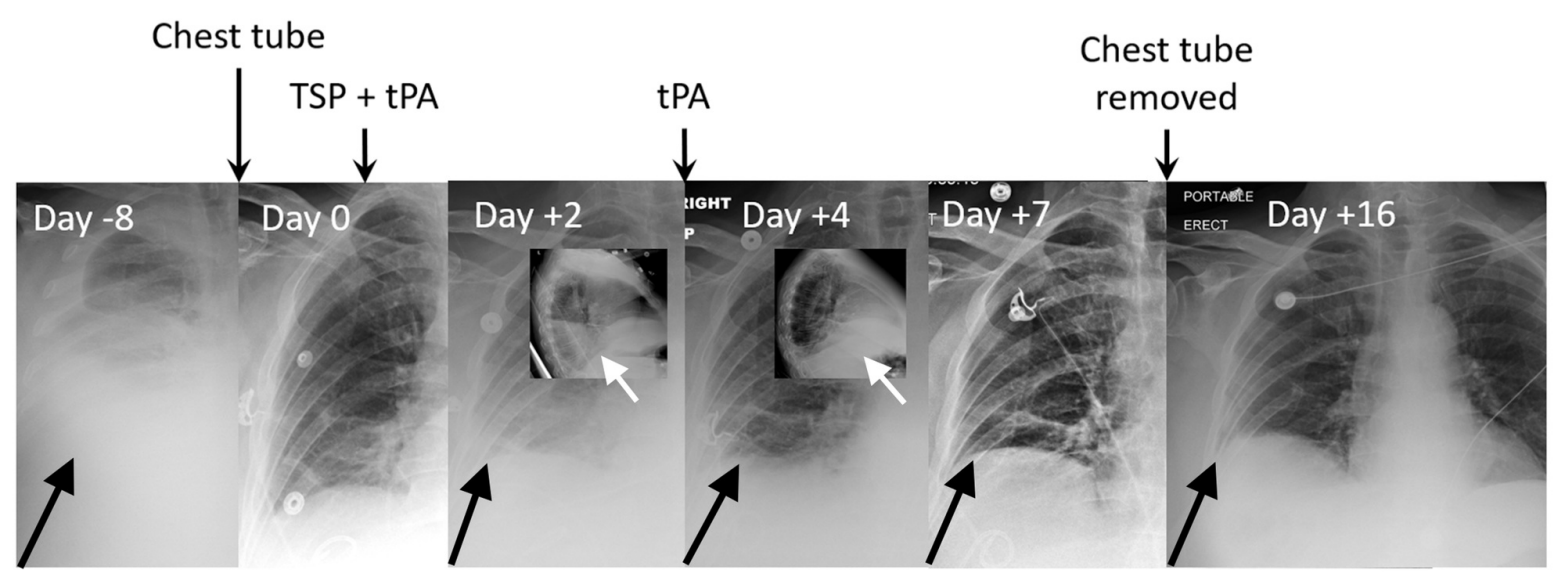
tPA after TSP for drainage of developing loculation. A 67-year-old man presented with hepatic hydrothorax (day -8). A pigtail chest tube was placed with output >2,000 cc/day. TSP with tPA, 6 mg, was given on day 0. Lateral chest x-ray on day +2 demonstrated accumulating loculation so tPA, 6 mg, was given on day +3. Lateral chest x-ray on day +4 demonstrated resolution of the effusion. Chest tube removed on day +8 with follow-up chest x-ray on day +16 showing resolution. TSP: talc slurry pleurodesis, tPA: tissue plasminogen activator
